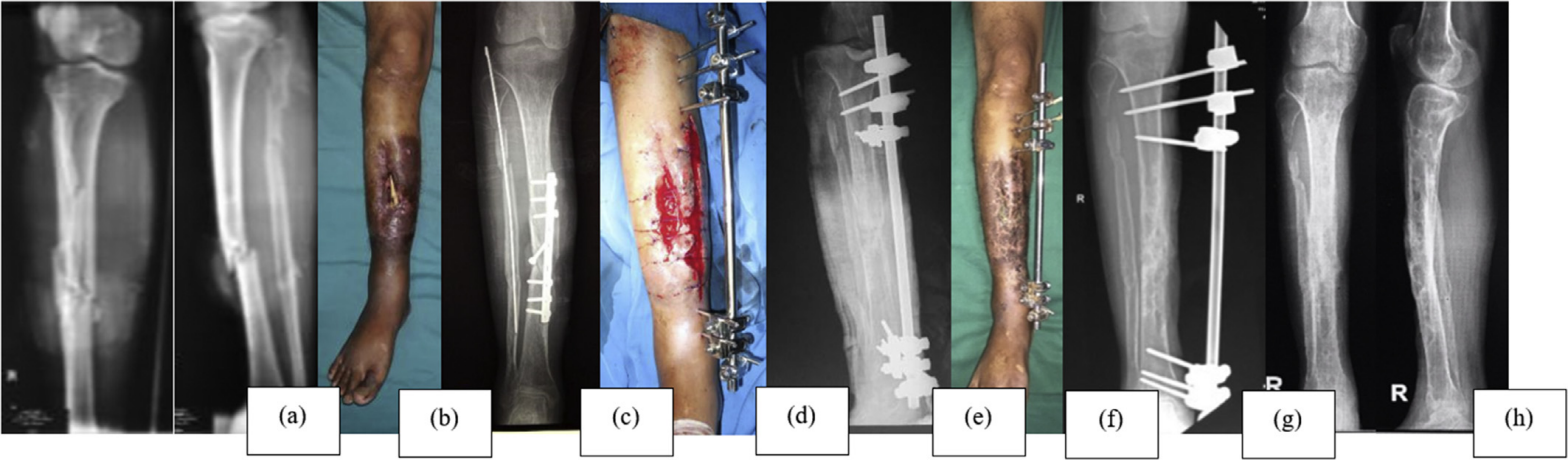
Case 3; a 38-year-old male with infected non-union of the right tibial shaft. (a) Initial anteroposterior and lateral X-ray of the tibia. (b) Anterior preoperative view of the right leg – exposed sequester. (c) Preoperative anteroposterior X-ray of the right leg. (d) Anterior postoperative view of the right leg – implant removal and external fixator application. (e) Postoperative anteroposterior X-ray of the right leg. (f) Anterior X-ray of the right leg, 9 months postoperatively – healed wound. (g) Anteroposterior view of the right leg, 9 months postoperatively – bony union. (h) Post-implant removal
